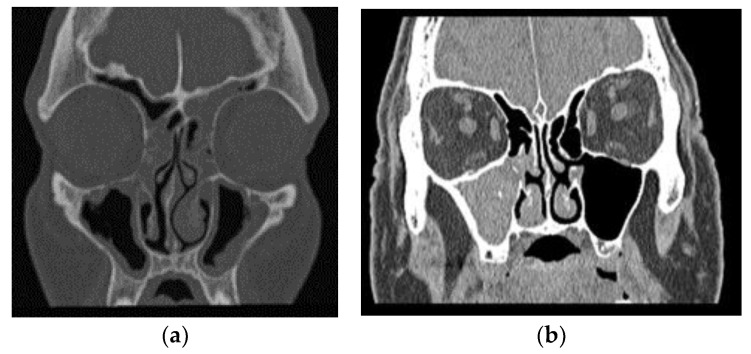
Typical CT scan of a patient with (a) chronic rhinosinusitis and (b) one sinus fungal ball.
